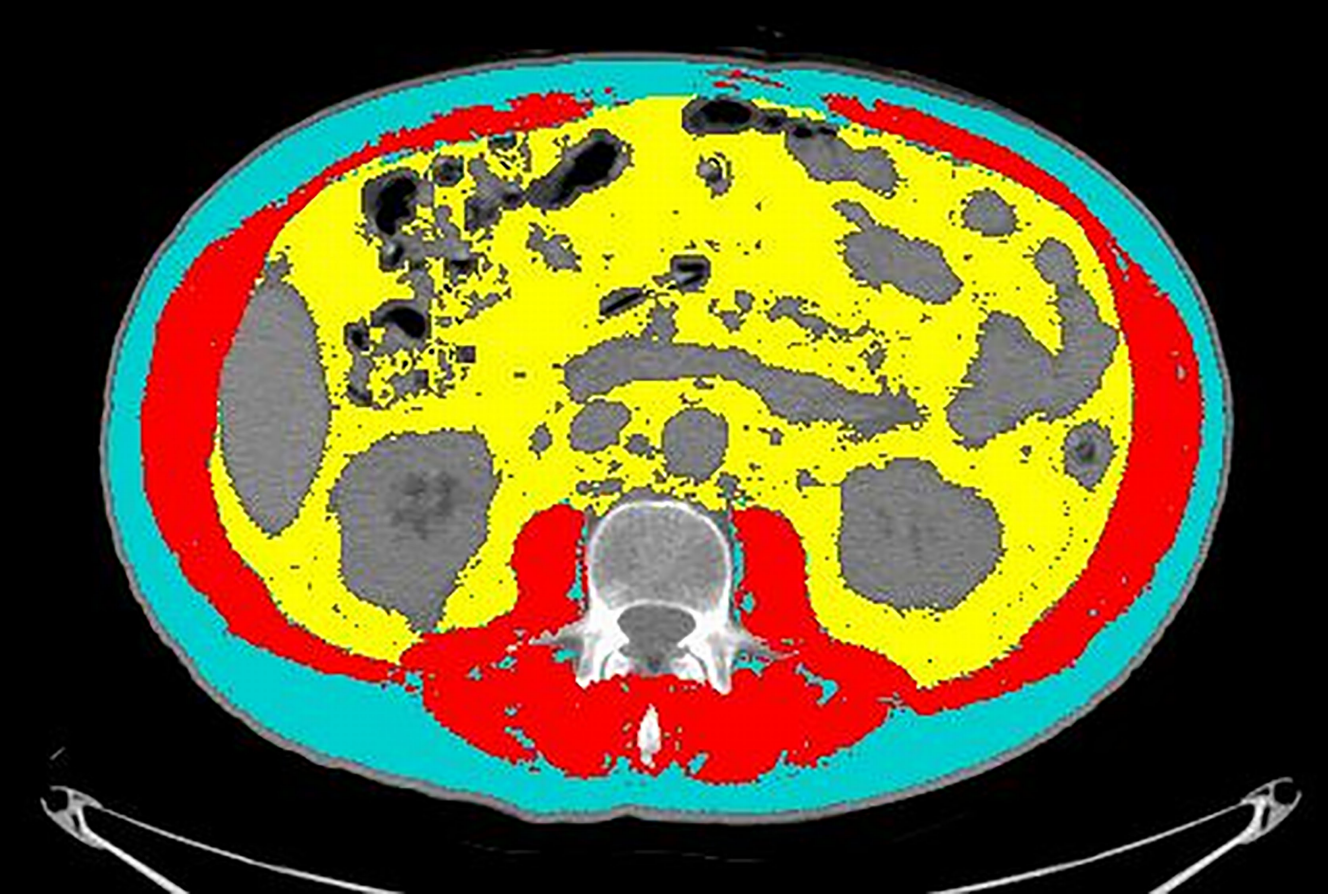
An example of segmentation of the CT image at the level of L3. Cyan indicates visceral adipose tissue (VAT) area; red indicates skeletal muscle area (SMA); yellow indicates visceral adipose tissue (VAT). Grey areas were excluded from measurements because they refer to abdominal organs (mainly liver, kidneys, and bowel).
